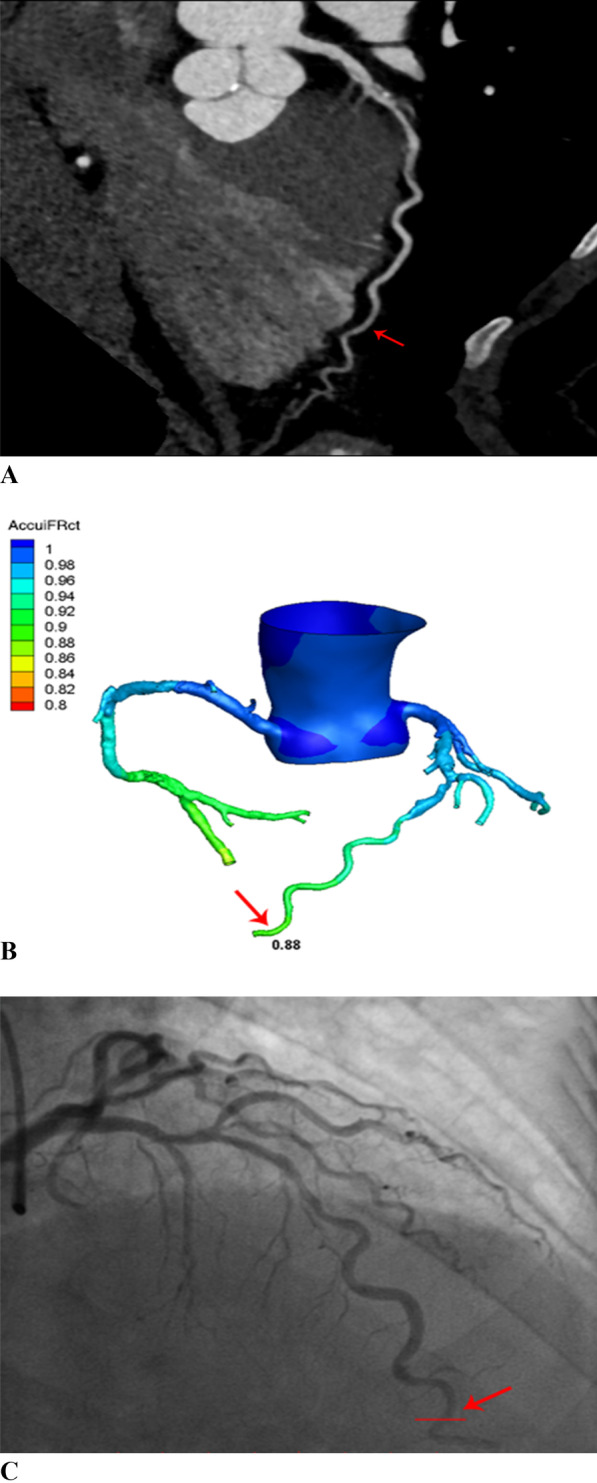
A CCTA demonstrating 41% stenosis at the portion of LAD (red arrow); B a computed AccuiFRct value of 0.88 (red arrow); C Coronary catheter angiography shows a stenosis (red arrow) with an iFR measurement of 0.86. iFR, instantaneous wave-free ratio; AccuiFRct, instantaneous wave-free ratio derived from computed tomography; CCTA coronary computed tomography angiography, LAD left anterior descending artery
